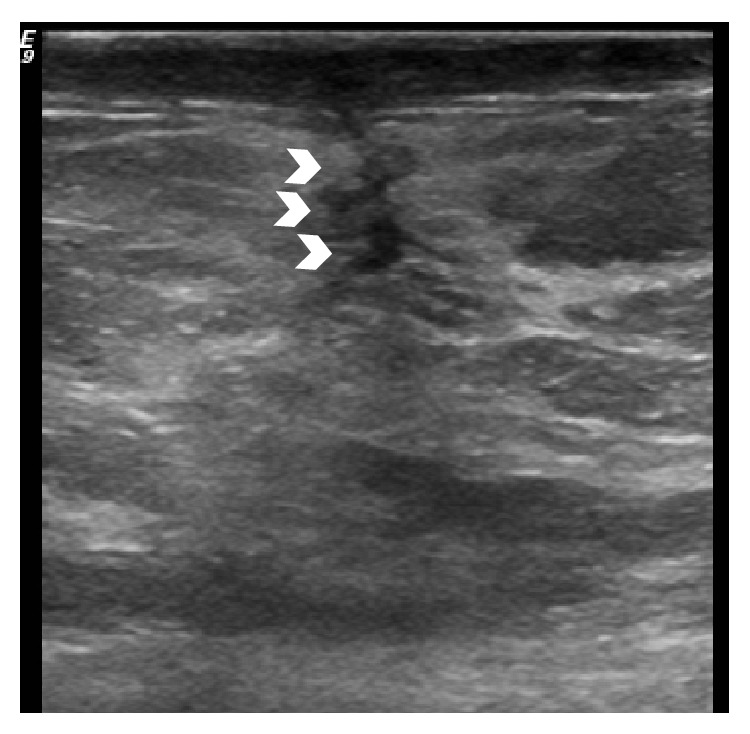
Follow-up ultrasound after surgical excision. A linear hypoechoic tract (arrowheads) can be seen at the site of surgery consistent with a surgical scar. No nodular soft tissue mass is identified to suggest residual disease.
